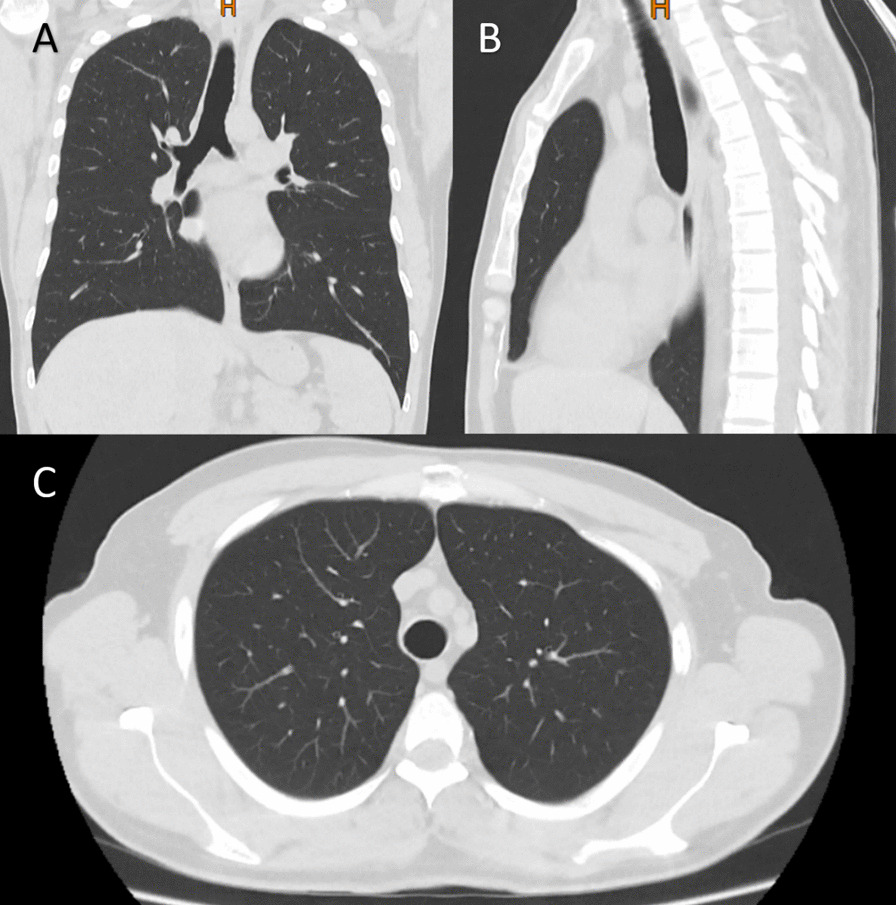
The computed tomography scan one month following surgical management of endotracheal tumor; A coronal view, B sagittal view, and C axial view
